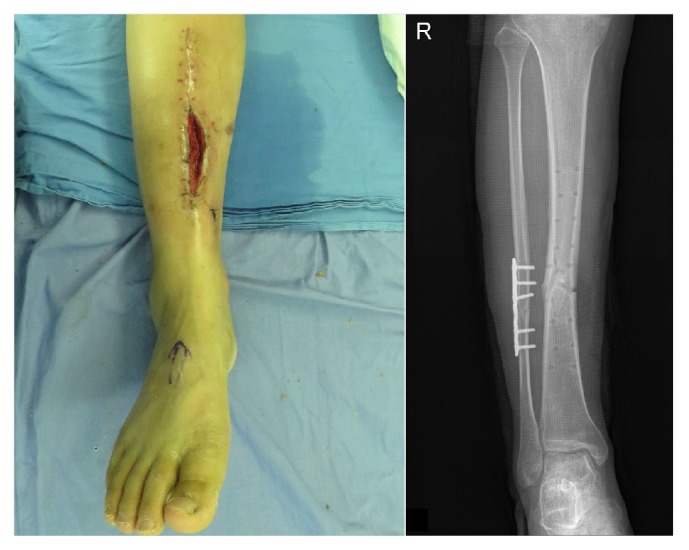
Case 17, a 33-year-old man with chronic osteomyelitis of the tibia. The left figure is the preoperative clinical photograph. The right figure is the preoperative anteroposterior X-ray photograph.
